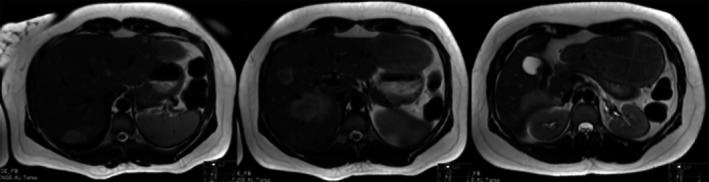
Abdominal CT: multiple hepatic adenomas.
